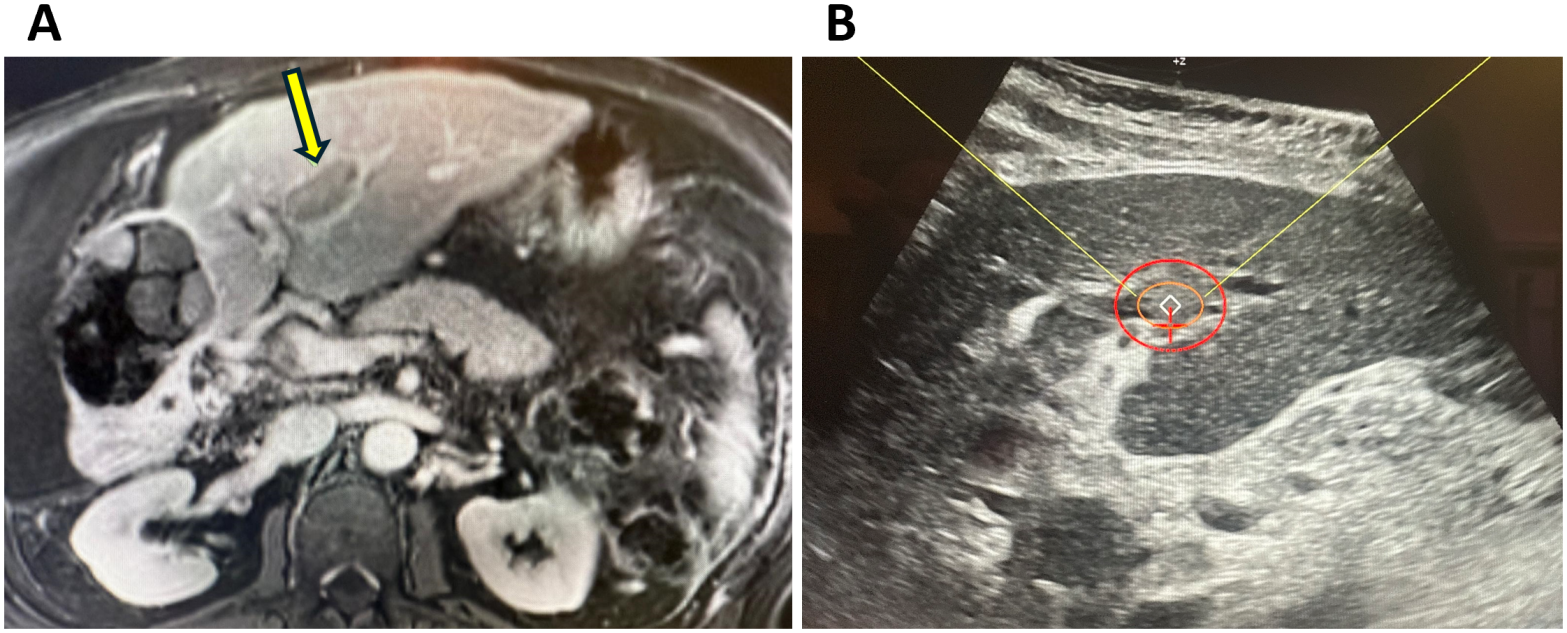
(A) Axial contrast-enhanced T1-weighted MR image after Y90 therapy showing the dominant HCC in the right lobe without any enhancement, consistent with near complete response according to mRECIST. The yellow arrow points to the portal vein tumor thrombus before targeting by histotripsy. (B) Preoperative ultrasound of the portal vein tumor thrombus being targeted by histotripsy, with the planning treatment volume (orange circle) surrounded by the treatment margin (red circle).
